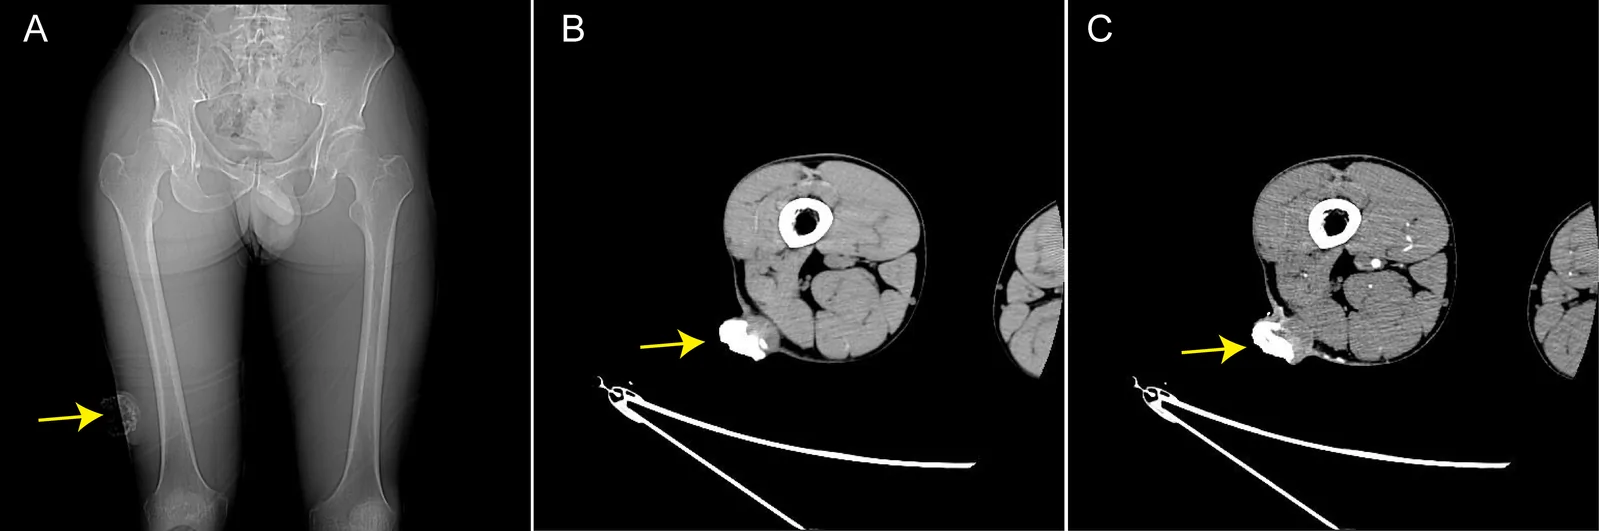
Radiographic and computed tomography (CT) evaluation of the lesion. (A) Scout view (topogram): A localized soft-tissue mass with internal stippled calcifications is observed on the lateral aspect of the right thigh (arrow). (B) Non-contrast CT: Axial slice showing a well-defined, exophytic lesion (arrow) with high-density calcified components. (C) Contrast-enhanced CT: Axial slice demonstrating the enhancement pattern of the lesion (arrow) and its relationship with the surrounding soft tissues.
